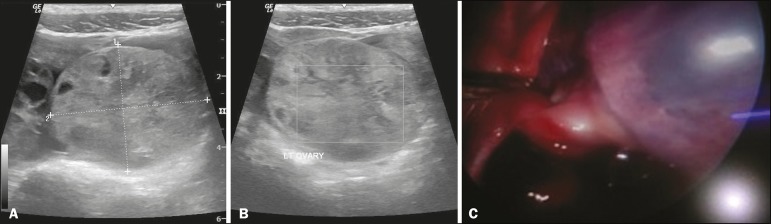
A: Ultrasound showing enlarged echogenic left ovary.
